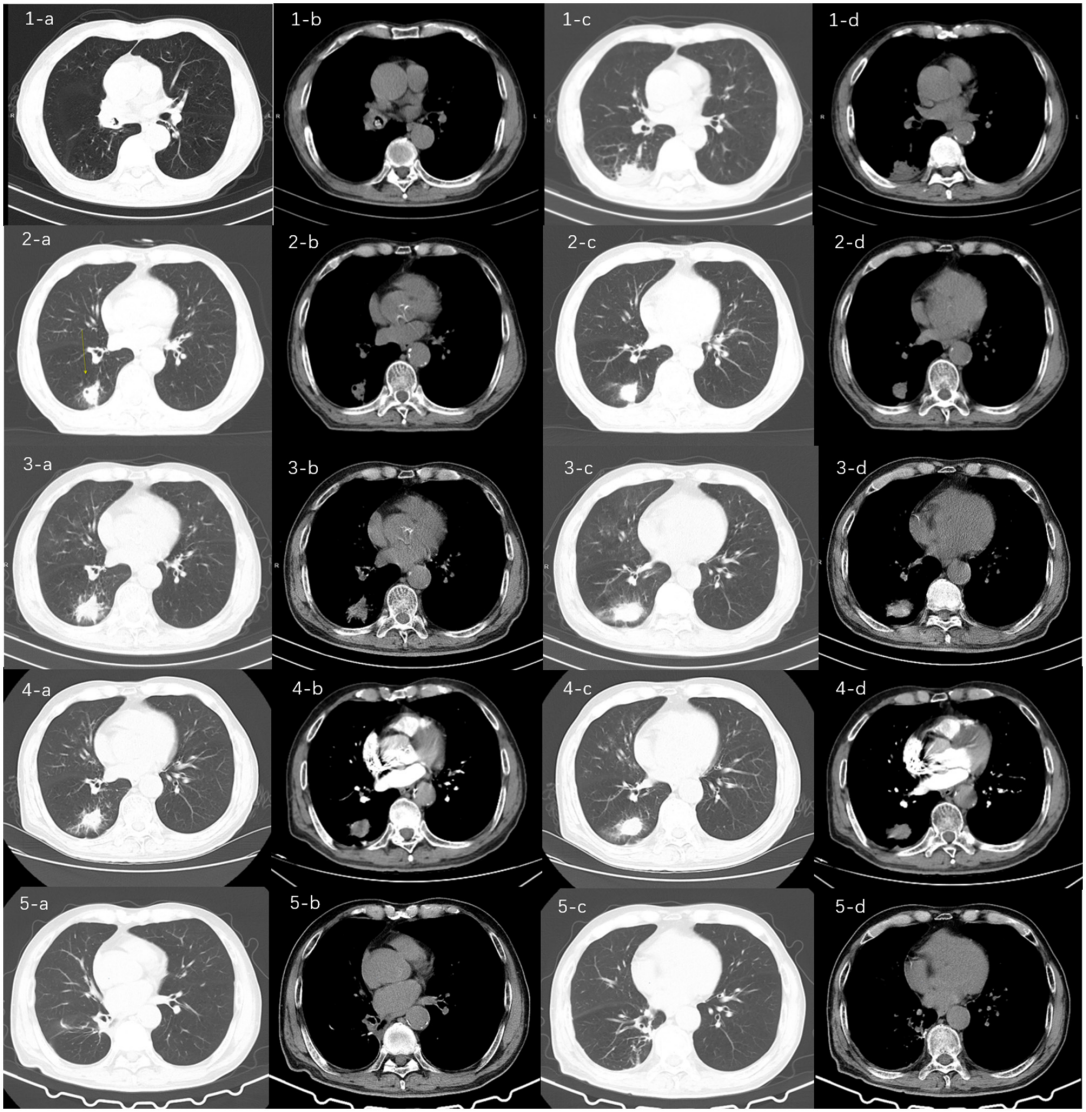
Chest computed tomography (CT) images of the patient over time. CT (1-a, b, c, d) at the time of initial admission (15 months prior) showed a dense shadow of the right middle bronchus, suggesting the possibility of a foreign body, along with partially solid infectious lesion in the right lower lobe. These findings prompted the first bronchoscopic intervention for foreign body removal; CT (2-a, b, c, d) from the second admission (11 months prior) showed a right lower lobe lesion with cavity formation, likely of infectious origin. The formation of a cavity is a characteristic progression of actinomycosis and was the primary reason for performing a CT-guided percutaneous lung biopsy to rule out malignancy or tuberculosis; CT (3-a, b, c, d) from the third admission (7 months prior) showed slight enlargement of the right lower lobe lesion and a reduction in cavity size. This mixed pattern of progression and regression under antibiotic therapy (moxifloxacin) raised diagnostic uncertainty, leading to the decision for bronchial artery embolization to manage life-threatening hemoptysis; and CT (4-a, b, c, d) from the fourth admission showed a lesion in the right lower lobe similar in appearance to the previous scan, with a further reduction in cavity size. The absence of significant bronchial artery dilation on CT angiography (not shown) and failure of medical hemostasis ultimately necessitated surgical resection, which provided the definitive diagnosis; CT(5-a,b,c,d) showed the patient's follow-up chest CT scan 2 months after surgery, which indicated no new lesions in the lungs and the patient's condition was stable.
